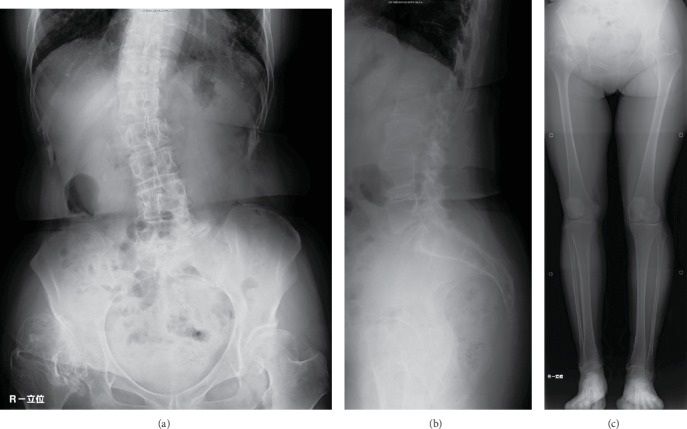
Anteroposterior (a) and lateral (b) radiograph of lumbar spine in standing. Anteroposterior radiograph of bilateral whole lower limbs in standing (c).
